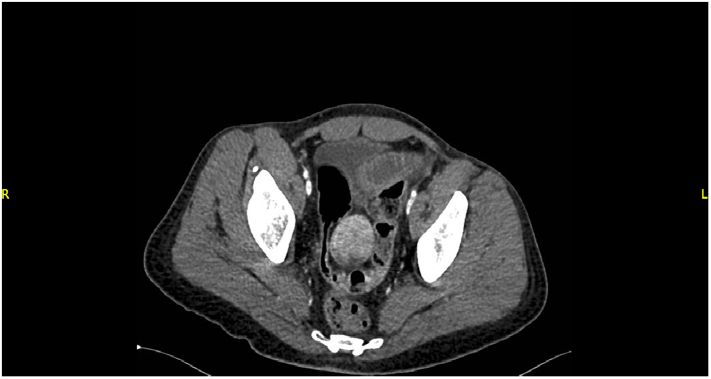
CT-angiogram showing a well-circumscribed, highly vascular mass (red arrow). (For interpretation of the references to colour in this figure legend, the reader is referred to the web version of this article.)
